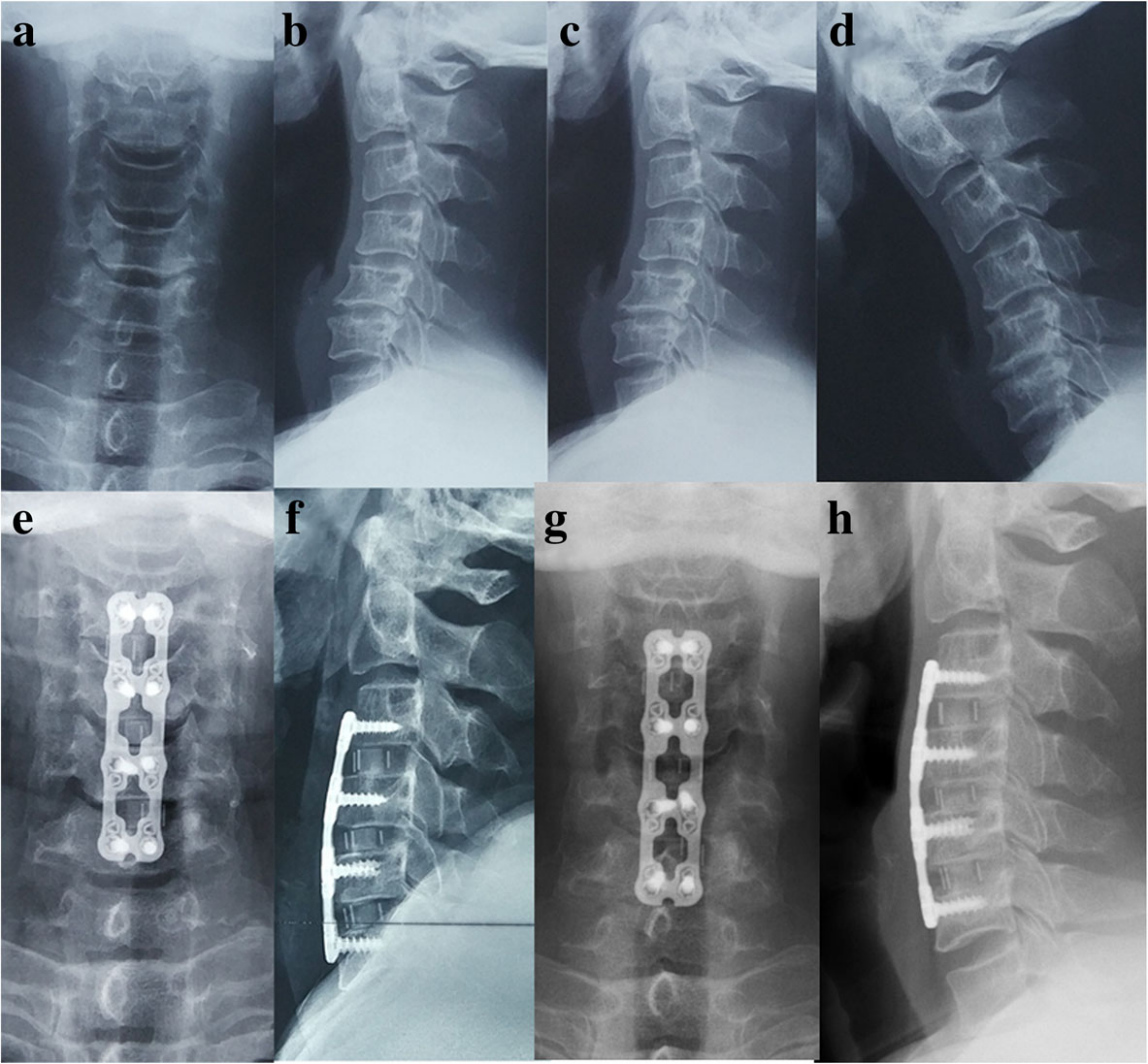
A 60-year-old male developed numbness in his two hands and weakness in his four extremities for 3 years. Preoperative radiographs showed that the sagittal alignment of the cervical spine was kyphotic (a–d). He was performed with 3-level ACDF and presented with axial neck pain without other surgery-related complications. After operation, his JOA scores improved from 9.4 preoperation to 14.7 postoperation and axial neck pain disappeared 2 months after surgery. Postoperative lateral and flexion-extension cervical radiographs showed that the cervical lordosis was improved (e, f) and the graft got bony fusion at 1-year follow-up (g, h)
